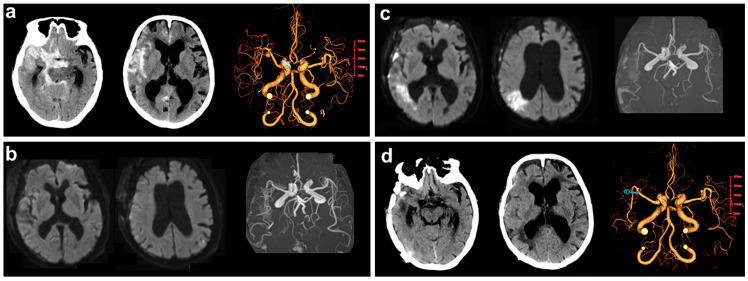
Imaging findings. (a) Head CT scans on admission in an 89-year-old patient with SAH showing ruptured right middle cerebral artery aneurysm and intra-sylvian hematoma. Although postoperative MR diffusion-weighted images and angiography performed on day 7 were unremarkable (b), new infarction and distal vasospasm were detected in the posterior trunk territory of the right MCA (c), suggestive of cerebral vasospasm and related DCI. (d) Follow-up CT scan on day 21.
